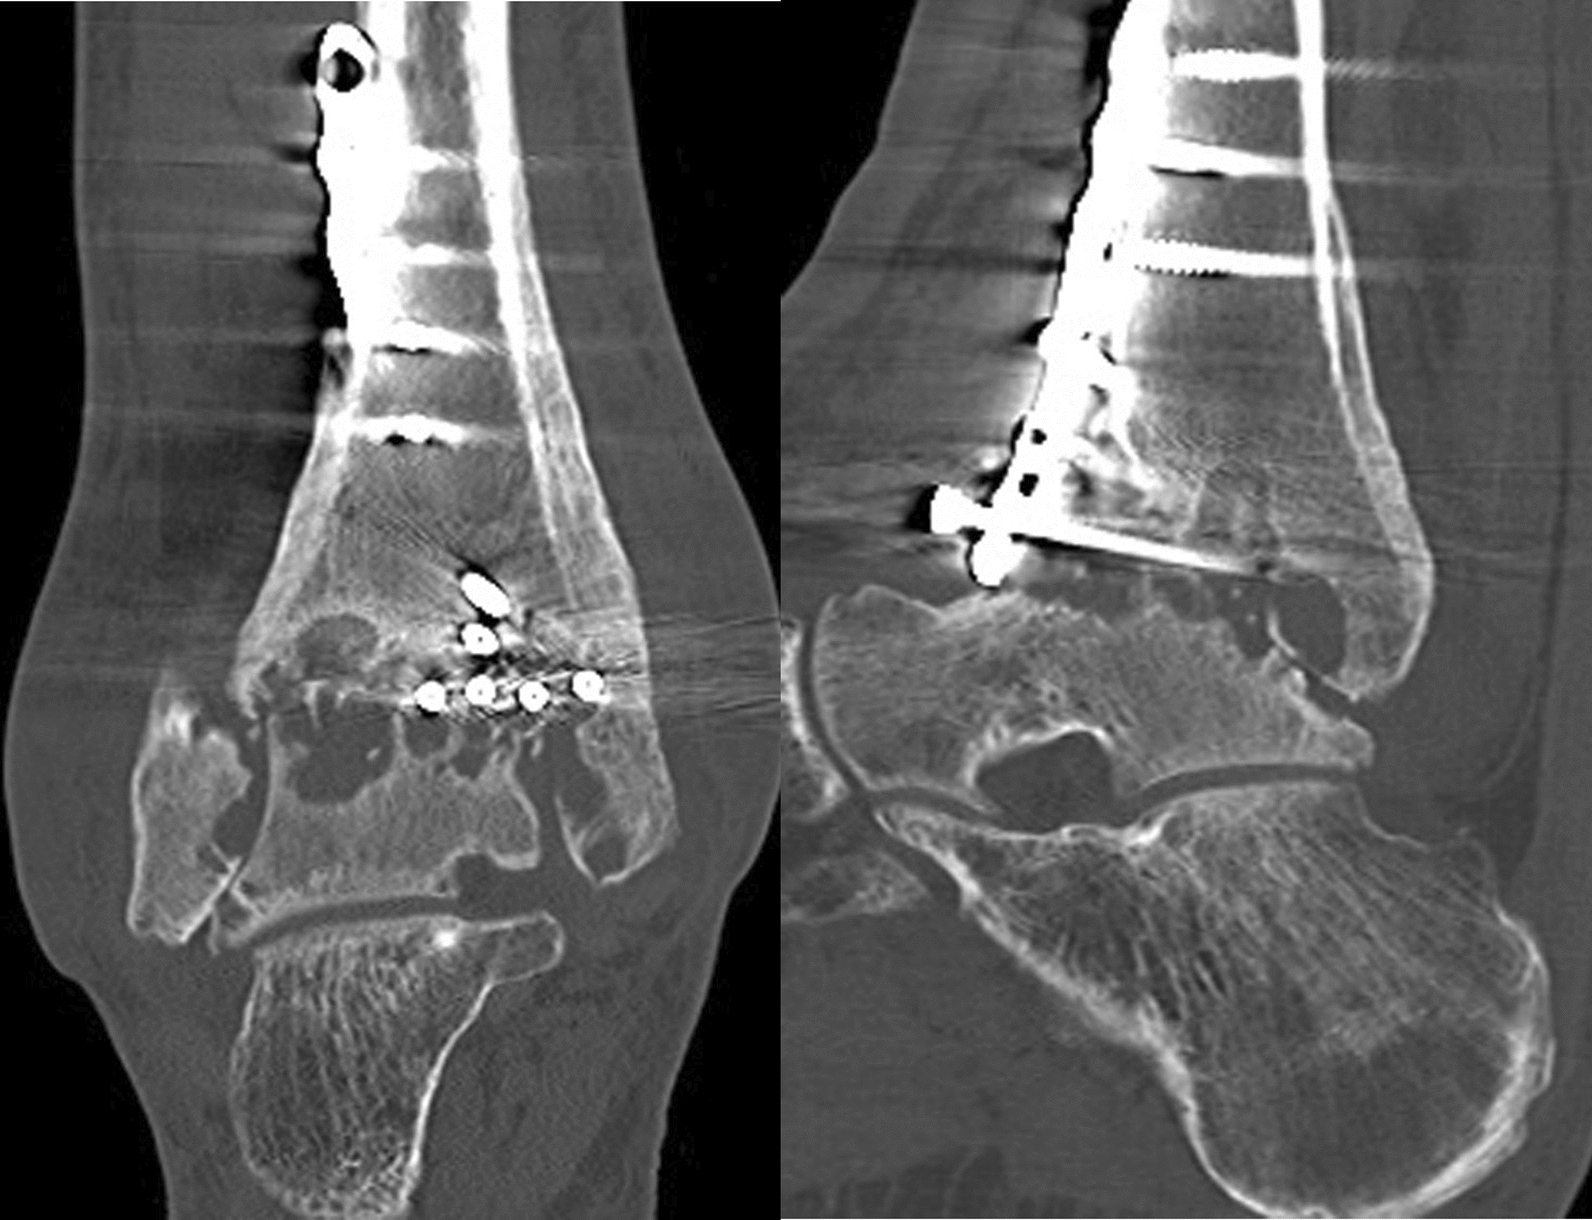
Plain computed tomography scan images of right ankle, presenting rapidly progressed joint destruction with extensive bone defects: (right) coronal view and (left) sagittal view
